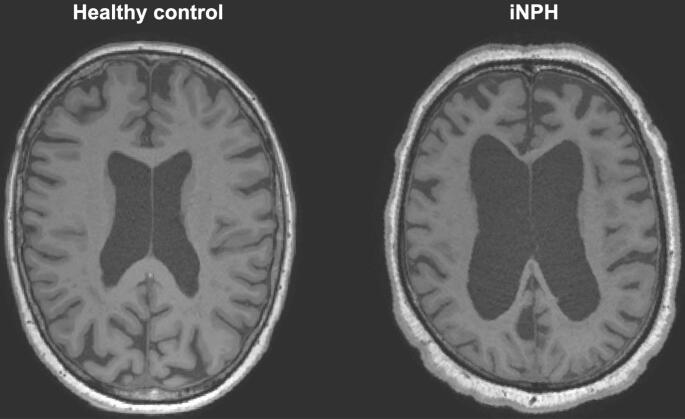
Representative T1w axial MRI images comparing ventricular morphology in a healthy control versus an iNPH patient. The iNPH patient (right) demonstrates significant ventricular enlargement compared to the healthy control (left).
